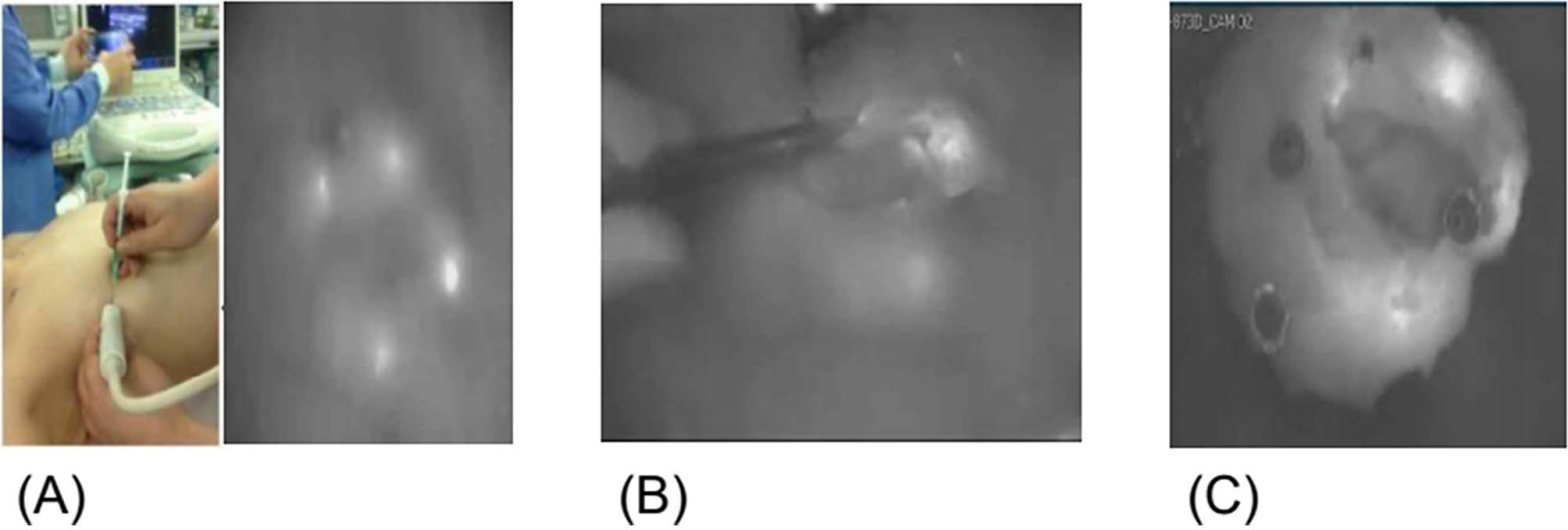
Localization by ICG injection (ICG injection into the target lesion under the guidance of ultrasonography). (A) The fluorescent agent is injected into 4–8 areas of the breast cancer resection surface using ultrasound before surgery. (B) During surgery, the surgeon finds and removes the fluorescent lesion using a near-infrared fluorescence camera. (C) After lesion removal, the completeness of the resection through a near-infrared fluorescent camera was confirmed.
